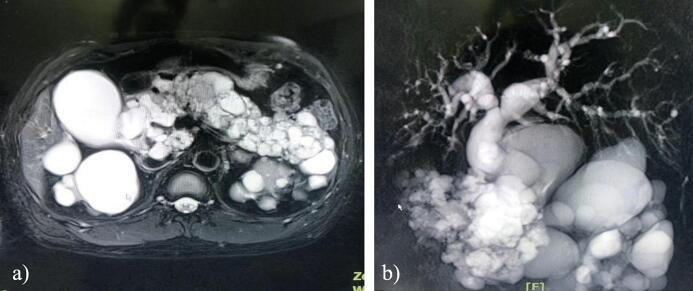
a) Contrast-Enhanced MRI Abdomen showed multiple cyst lesions in the pancreas and both kidneys, b) MRCP showed multiple pancreatic cysts with dilatation of the biliary tree.
